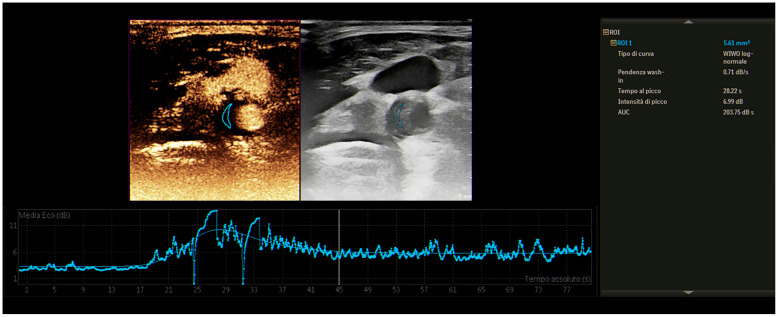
Case-based extraction of dynamic signal behavior using bolus-based wash-in/wash-out analysis.* Representative WI/WO TIC analysis of an internal carotid artery plaque. Axial dual-display images show contrast-specific imaging alongside the corresponding grayscale B-mode image. The curvilinear blue ROI is positioned within the deeper portion of the plaque, avoiding luminal contamination. TIC analysis, performed after automated in-plane motion correction, depicts bolus passage fitted using a log-normal model, yielding semi-quantitative parameters including wash-in slope, time to peak, peak intensity, and area under the curve. Although the curve remains visually asymmetric, fitting is performed in the logarithmic time domain. The thicker oscillating blue trace represents the raw frame-by-frame contrast-enhanced ultrasound signal dominated by microbubble backscatter, whereas the smooth blue curve corresponds to the fitted model. The vertical white marker indicates the cine-loop time point corresponding to the displayed B-mode and contrast-specific ultrasound frame.
